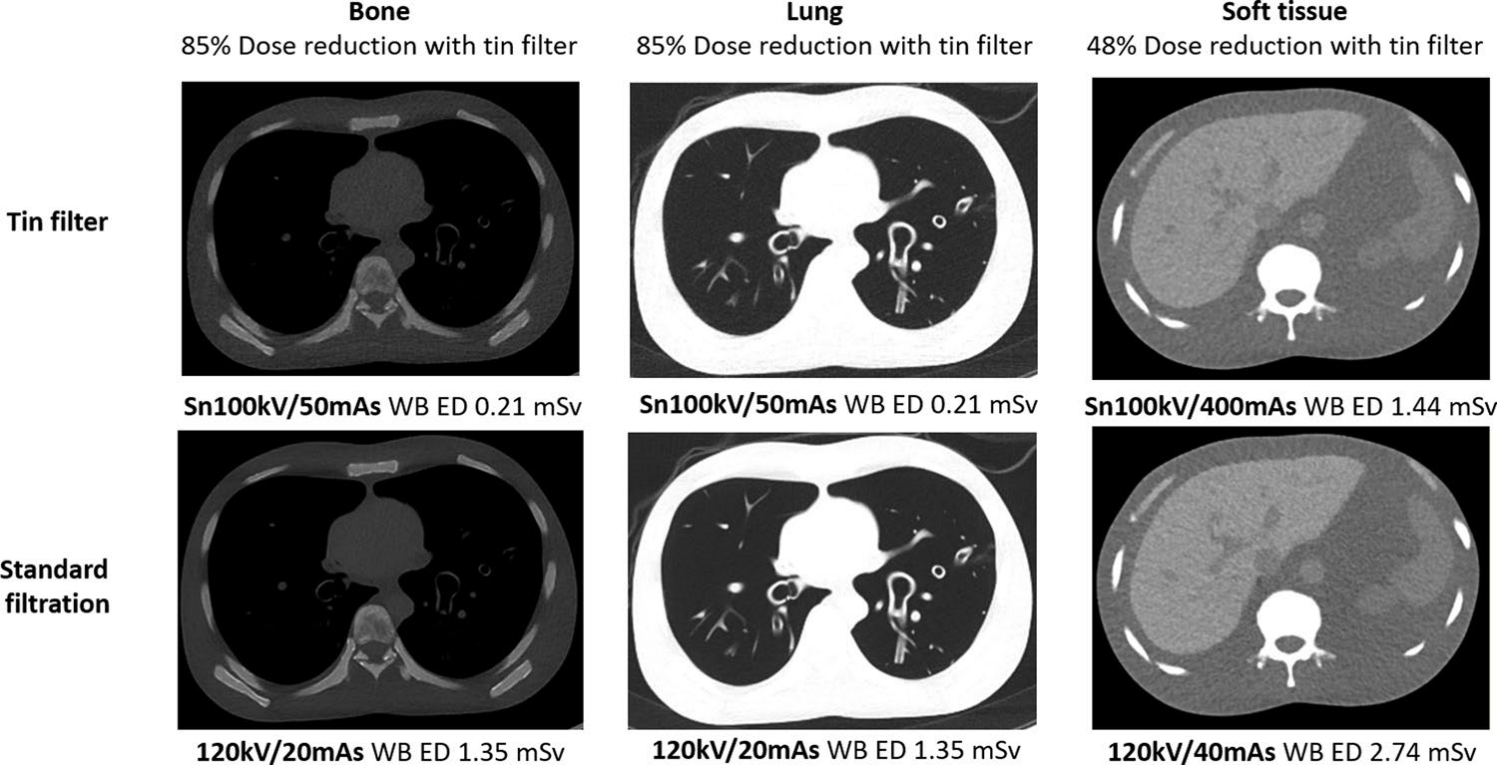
Image quality in bone, lung and soft tissue for CRS and reduced-dose tin filter protocols. ED = effective dose; mAs = milliampere seconds; Sn100kV = tin filter applied to X-rays generated using a peak tube voltage of 100 kV; Sn140kV = tin filter applied to X-rays generated using a peak tube voltage of 140 kV; WB = whole-body
